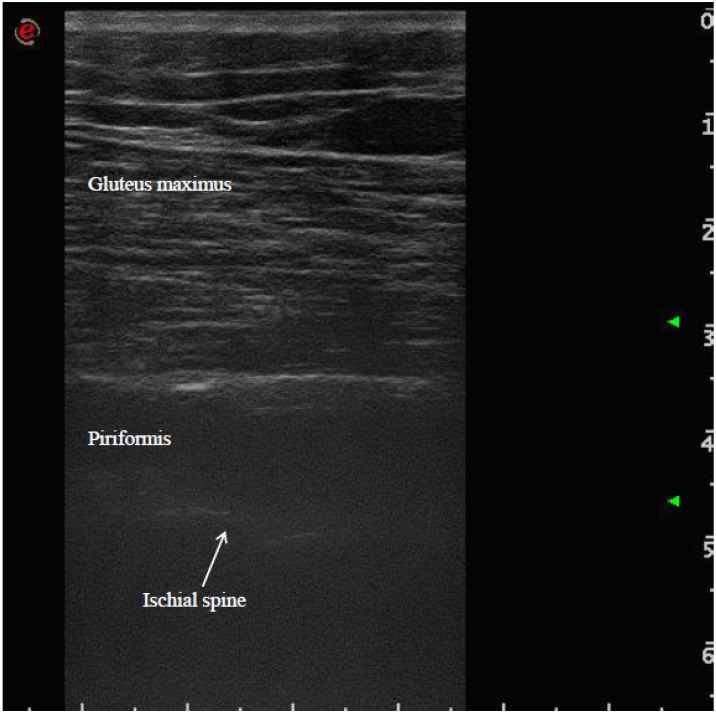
Ultrasound identification of the piriformis muscle between gluteus maximus muscle and ischial spine.
